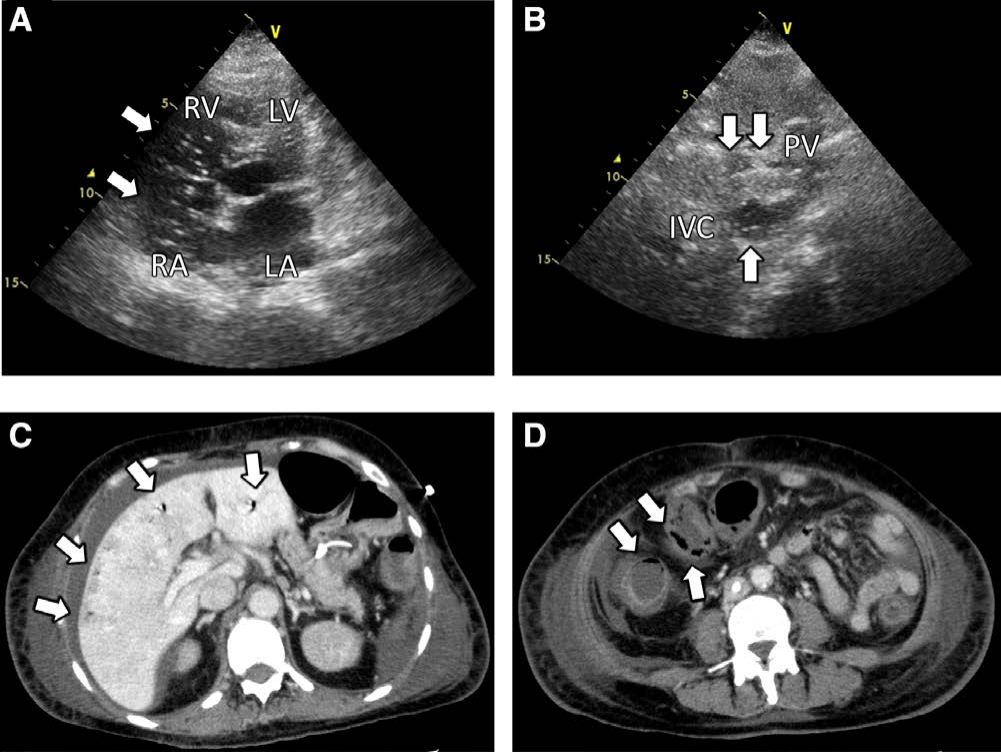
(1A, white arrows) A large number of bubbles were observed flowing from the right atrium towards the right ventricle. (1B, white arrows) Similar echogenic images were observed flowing in the portal vein and inferior vena cava. (1C, white arrows) Contrast-enhanced computed tomography of the abdomen was performed and revealed gas in the intrahepatic portal vein, as well as (1D, white arrows) poor contrast and intramural emphysema in the colon wall. IVC = inferior vena cava, LA = left atrium, LV = left ventricle, PV = portal vein, RA = right atrium, RV = right ventricle.
